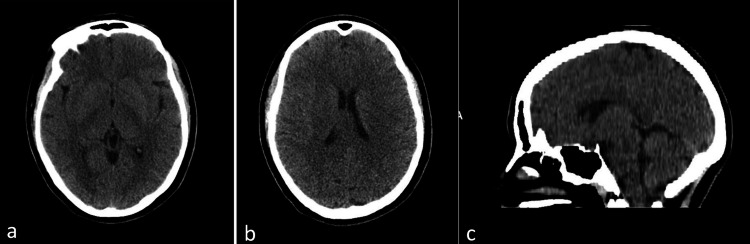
Computed tomography (CT) of the Head Without Contrast Radiology report impression: no evidence of acute intracranial process. (a) Axial/transverse view, (b) axial/transverse view, and (c) sagittal view
